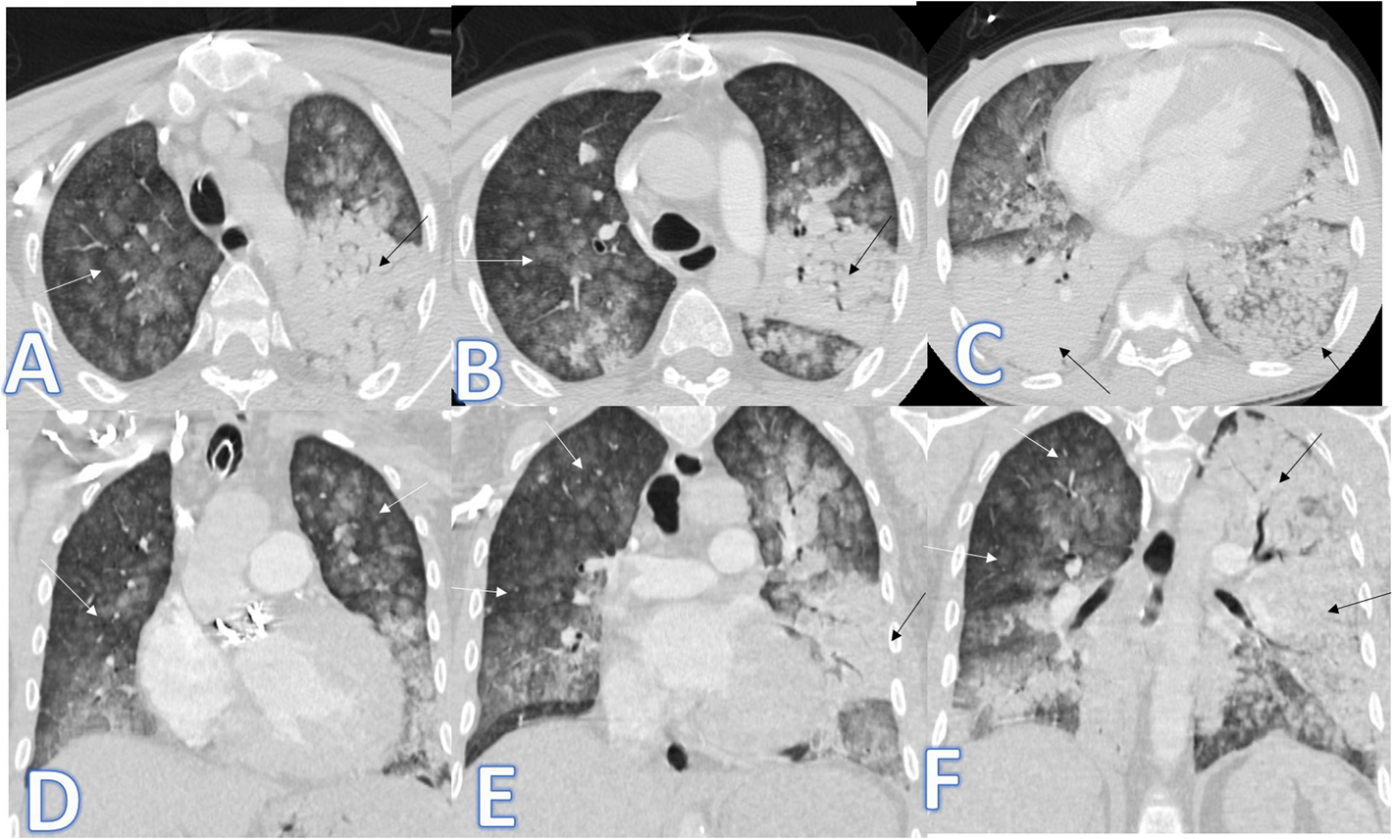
A 32-year-old male patient with ESRD on dialysis presented with fever and cough. CT showed bilateral high-density ground glass centrilobular nodules (white arrows) due to metastatic pulmonary calcification occurring in renal failure. Associated left lung consolidative patches with air bronchogram (black arrows). The diagnosis was metastatic calcification with superadded lung infection
